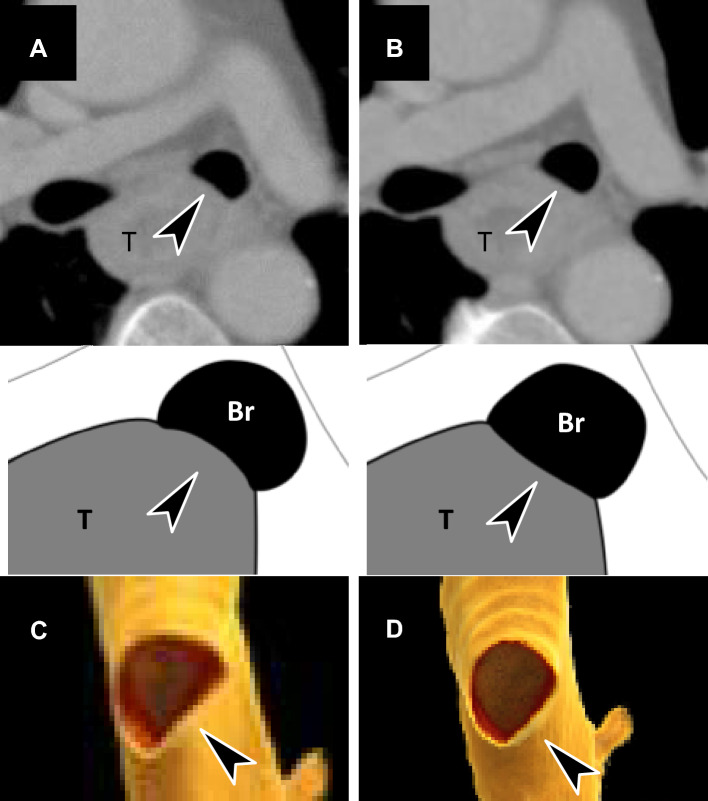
Axial and volume rendering images of a resectable locally advanced esophageal carcinoma (LAEC) on four-dimensional ventilation CT (4DCT) in a 63-year-old man (a–d). This is a case of suspected left bronchial invasion (arrowhead) examined by 4DCT; bronchial membranous part is compressed by the tumor in the maximum expiratory phase (a, c), and the finding is released in the maximum inspiratory phase (b, d), suggesting resectability for bronchial invasion. Supplemental Material 1 corresponds to this movie. *T* tumor, *Br* bronchus
